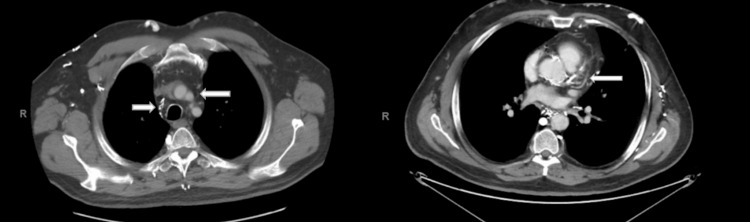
Fibrosing mediastinitis in a 56-year-old male; diffuse infiltrative mediastinal tissue (indicated by arrows) with calcifications. Contrast-enhanced computed tomography of the chest. Axial view.
